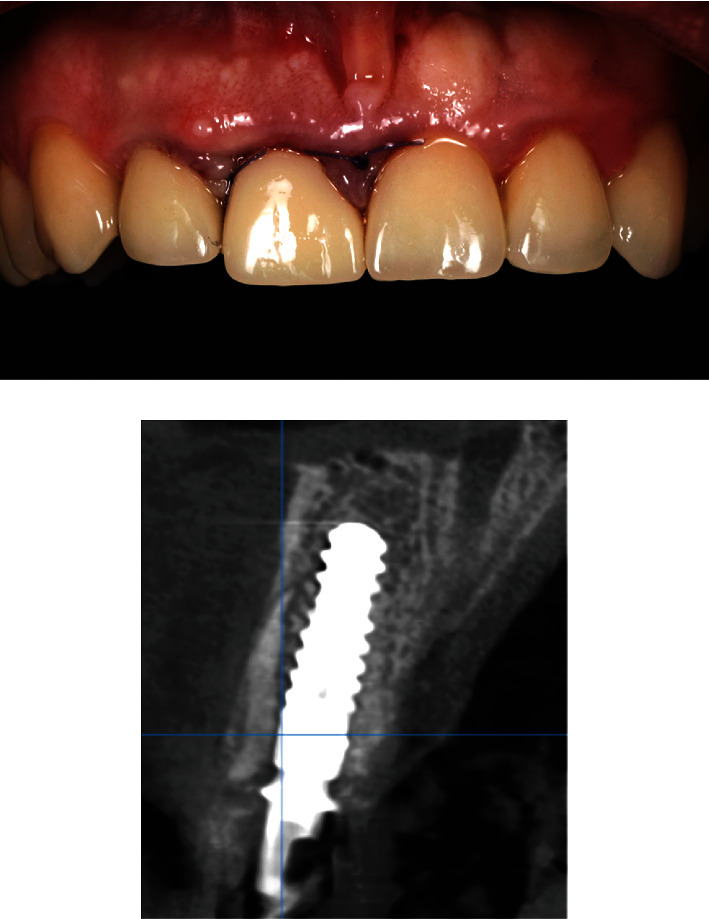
Immediate post-operative with fitted immediate crown and CBCT.
